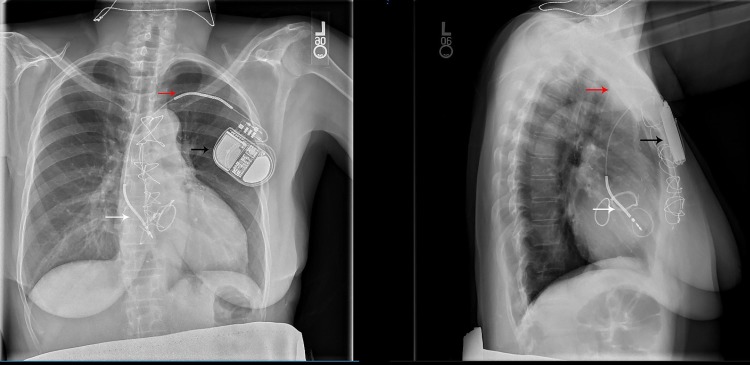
Posterior-anterior chest x-ray taken in 2017 Note the position of the right ventricle lead (white arrow) and coil (red arrow). The generator (black arrow) is flipped.
